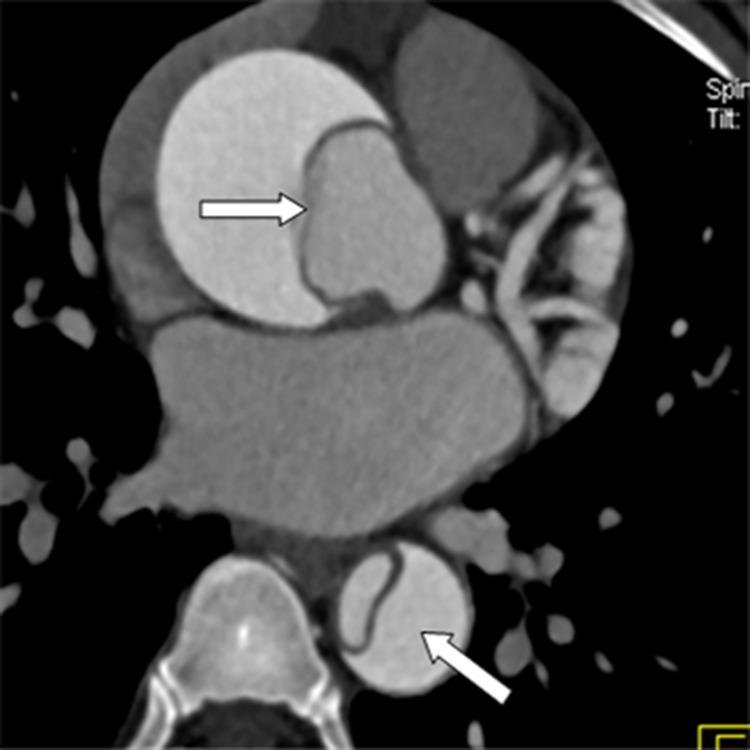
Single-line type of the intimal flap on an axial CT image.
